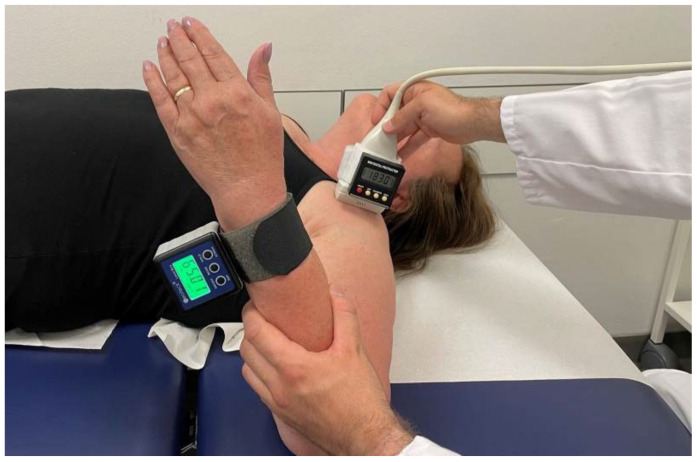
Sonographically assisted indirect measurement of humeral torsion. The angles determined are each given a positive sign if the corresponding protractor is inclined inward/caudal during the measurement and a negative sign if the protractor is inclined outward/cranial during the measurement. As the corresponding protractor attached to the transducer is inclined outward/cranial in this case, a negative sign must be given to this determined angle. As also the vertically positioned forearm protractor was zeroed on the horizontal table plane before attachment, its measured angle has to be subtracted from 90° to obtain the actual forearm angle. In this case, the forearm protractor displayed 65.01°, which results in 24.99° as actual forearm angle (90°–65.01°). All values were rounded for further calculation.
